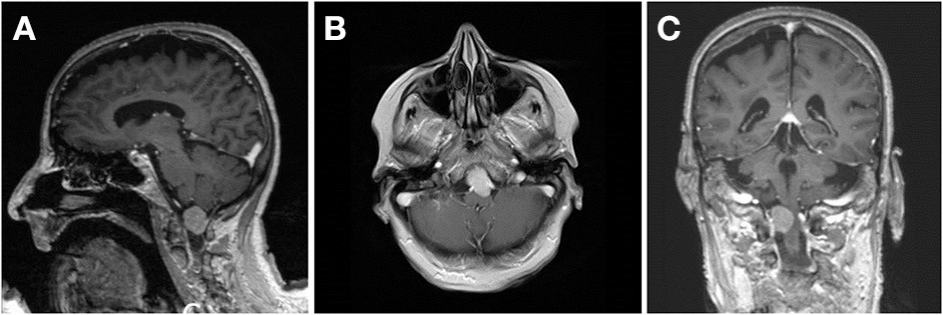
Preoperative sagittal (A), coronary (B) and transverse (C) contrast-enhanced T1-weighted image of case 4 depicting a lateral craniocervical meningioma growing anterior and posterior from the denticulate ligament.
